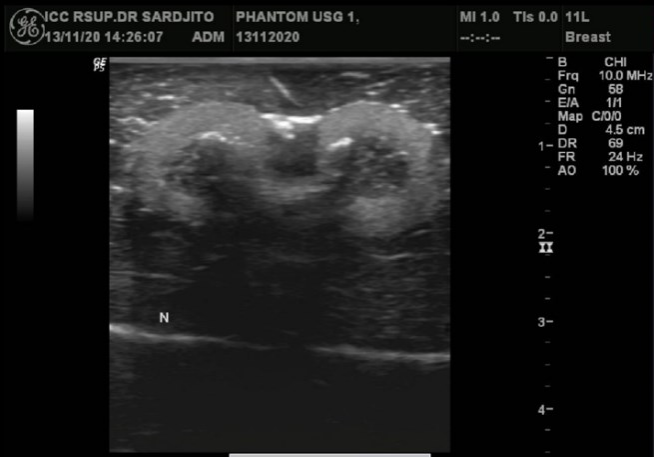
Ultrasound images of two artificial solid tumors made of olive and carrot pieces to represent oval inhomogeneous lesions. Olive flesh appeared as a mildly hyperechoic area at the edge of the lesion, while carrot pieces appeared as a hypoechoic area in the middle portion with a posterior shadow and several small linear hyperechoic areas.
